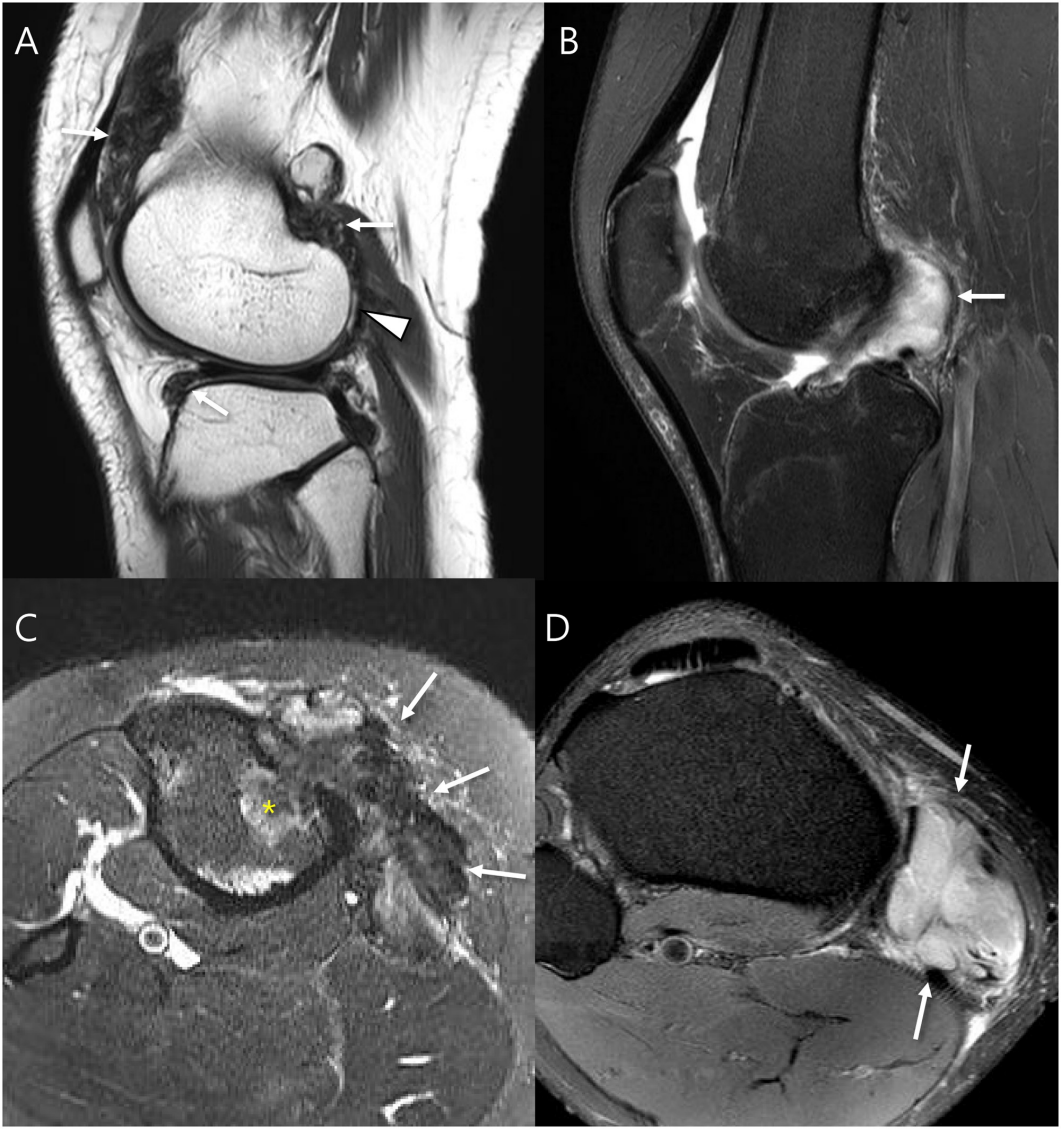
MRI features regarding disease subtypes. (A) Sagittal T2-weighted image of a 24-year old woman with intraarticular D-TSGCT shows soft tissue masses that are very low signal intensity located in knee joint (arrows) showing infiltrative margin and containing granular internal hypointensity without visible peripheral hypointensity. Note that the lateral femoral condyle cartilage injury is combined (arrowhead). (B) Sagittal T2-weighted image with fat suppression of a 26-year old woman with intraarticular L-TSGCT shows soft tissue mass at the posterior femoral recess (arrow). Note that the mass shows a circumscribed margin and contains a speckled internal hypointensity with peripheral hypointensity. (C) Axial T2-weighted image with fat suppression of a 40-year old woman with extraarticular D-TSGCT shows soft tissue masses that are very low signal intensity at the insertion of the pes anserinus conjoined tendon (arrows) presenting the multinodular masses with infiltrative margin with bone invasion (*) and containing granular internal hypointensity (arrows) without visible peripheral hypointensity. (D) Axial T2-weighted image with fat suppression of a 30-year old man with extraarticular L-TSGCT shows soft tissue masses along the pes anserinus conjoined tendon (arrows) presenting a circumscribed margin and containing a speckled internal hypointensity (arrows) with peripheral hypointensity.
